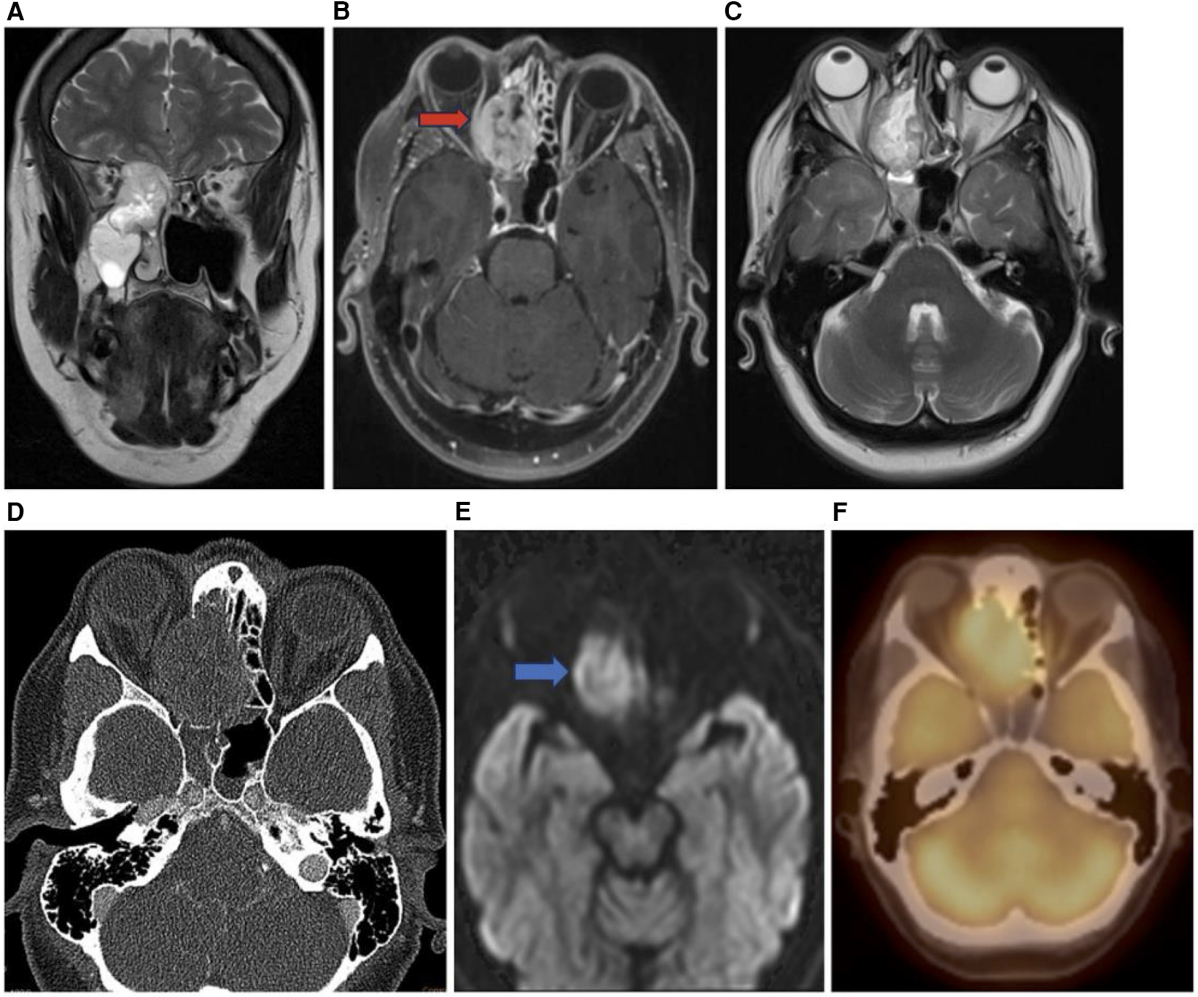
Sinonasal melanoma. Coronal T2 (A), axial T1 + C (B), axial T2 (C), axial CT bone (D), axial DWI (E), and axial PET (F) images showing an enhancing sinonasal melanoma centered in the right nasal cavity (red arrow) extending into the adjacent right orbit and into the anterior cranial fossa demonstrating diffusion restriction (blue arrow) and PET activity.
